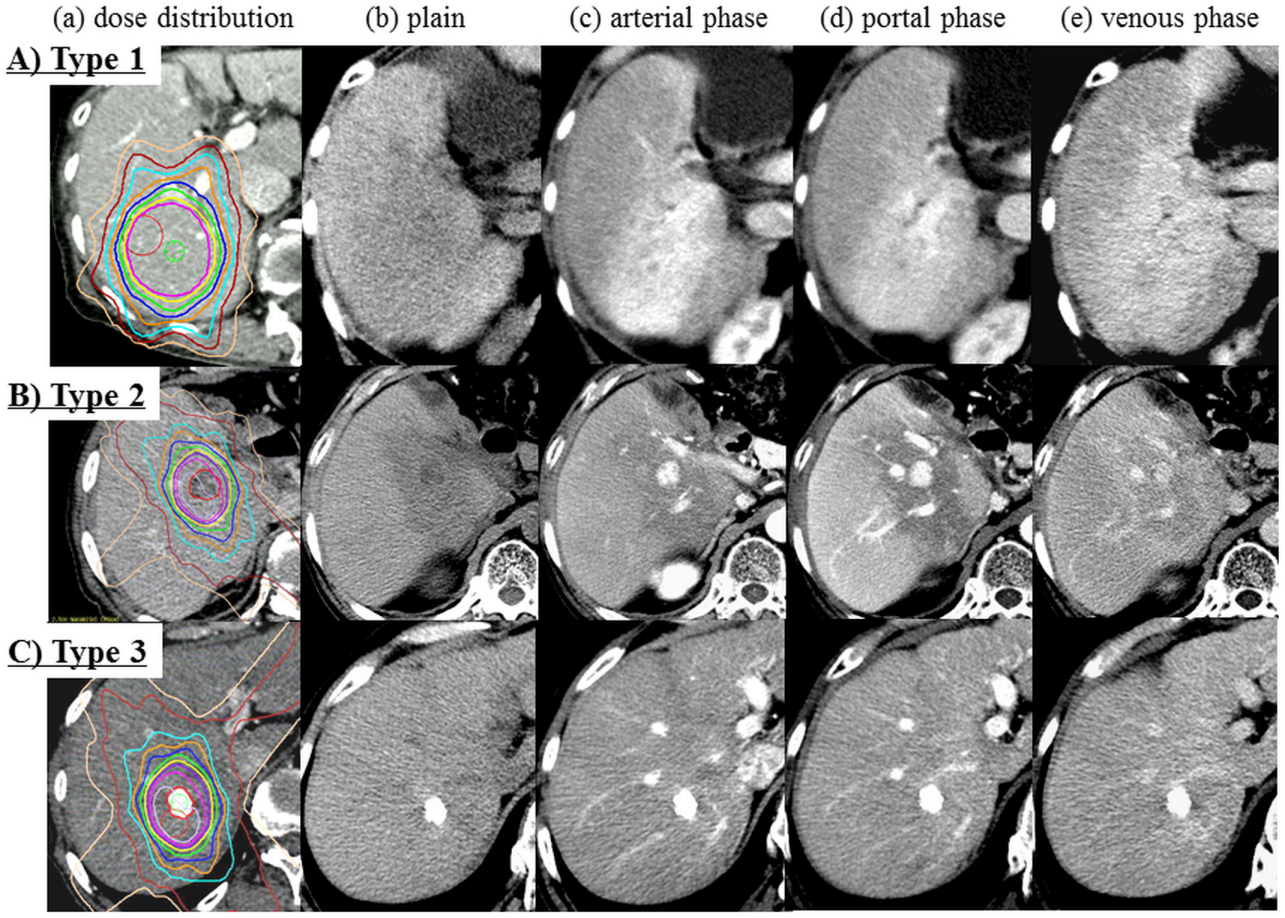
The dynamic CT appearance of focal liver injury following SBRT for HCC was classified into 3 types. A) Type 1 (case 12, 10 months following SBRT). a) Dose distribution b) Plain c) Arterial phase d) Portal phase e) Venous phase. Hypodensity in plain CT and hyperdensity in all enhanced phases. B) Type 2 (case 39, 2 months following SBRT). a) Dose distribution b) Plain c) Arterial phase d) Portal phase e) Venous phase. Hypodensity in the arterial and portal phases and isodensity in the venous phase. C) Type 3 (case 51, 2 months following SBRT). a) Dose distribution b) Plain c) Arterial phase d) Portal phase e) Venous phase. Isodensity in the enhanced phases.
